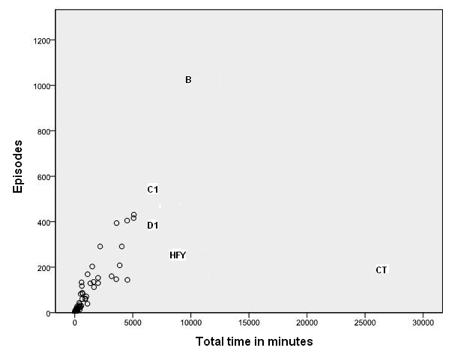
Number of episodes versus total time logged on to the discussion forum for all participants. This shows Caretaker (CT) (with over 25,000 minutes logged on) and Butterfly (B) (with many short episodes) as outliers, as well as Discussant 1 (D1), Crisis-oriented 1 (C1), and Here for you (HFY)
